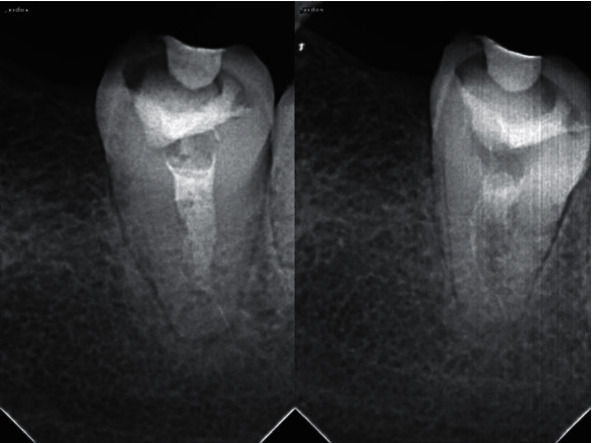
Annual follow-up radiographs demonstrating movement of the fractured segment into the root canal.
